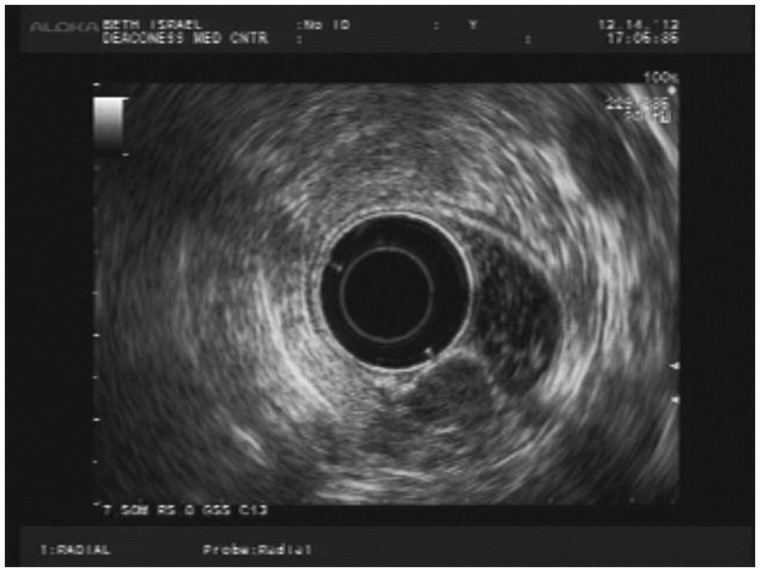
Endoscopic ultrasound image of the mass arising from the *muscularis propria* (EUS layer 4)
